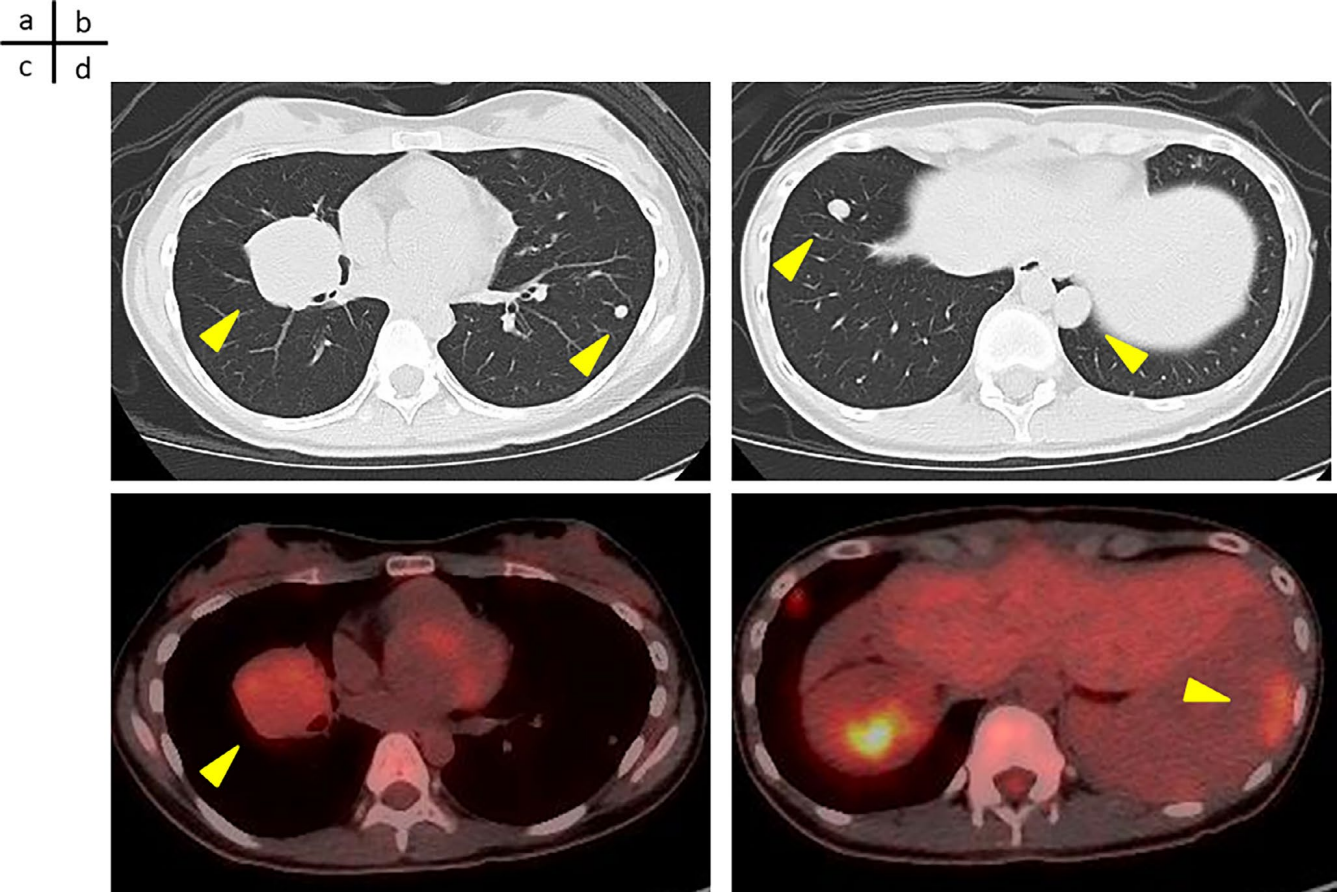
Plain chest CT scan and PET‐CT scan 1 year and 5 months after referral. (a) Plain chest CT showing a mass with a mild FGD accumulation near the right hilum (arrow). (b) Plain chest CT showing an enlarged nodule in the left lung at the time of presentation (arrow). (c) PET‐CT reveals a mass with a mild FGD accumulation near the right hilum (arrow). (d) PET‐CT showing peritoneal dissemination (arrow).
